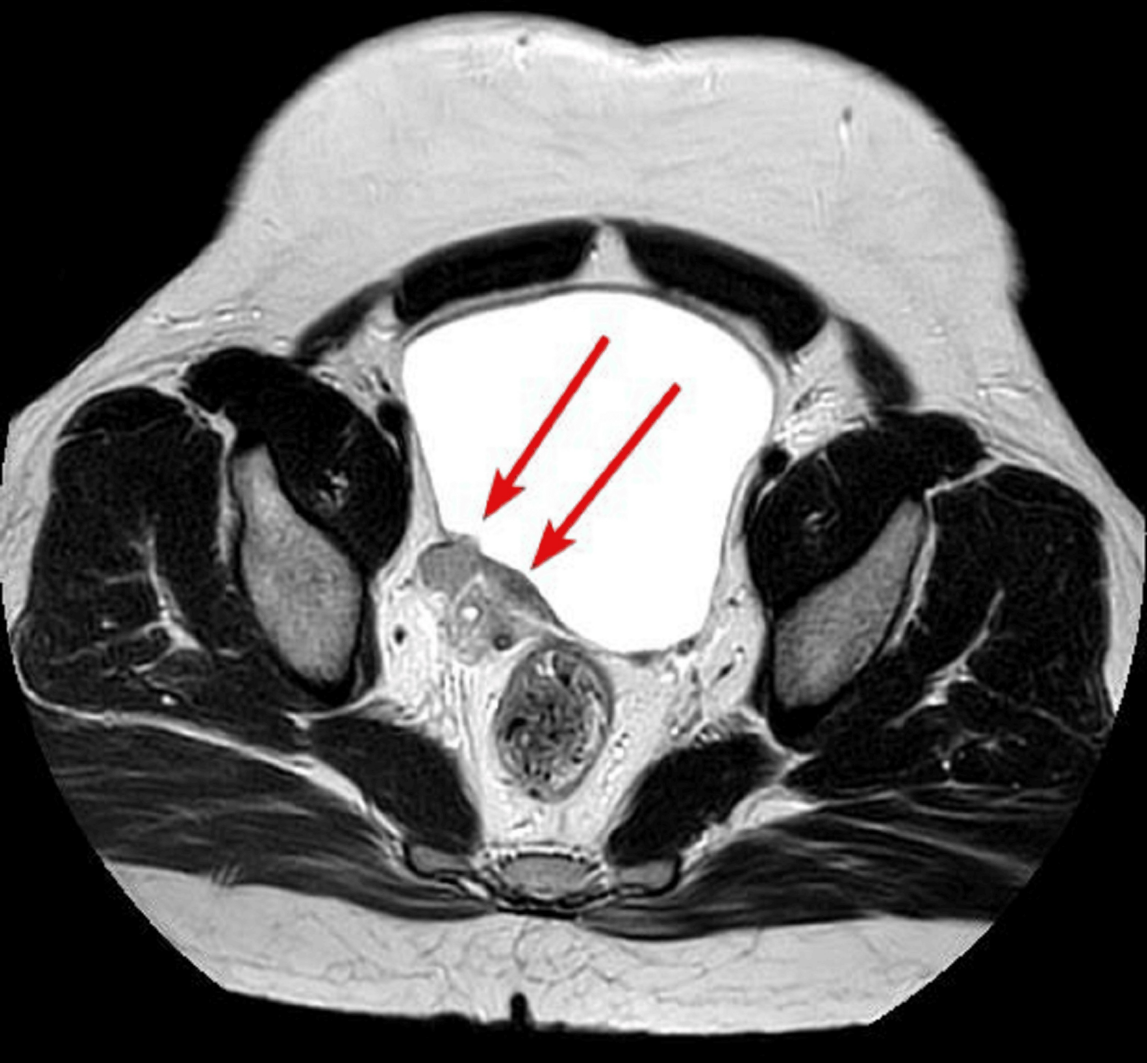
Bladder wall lesion visualized on MRI T2 images.
